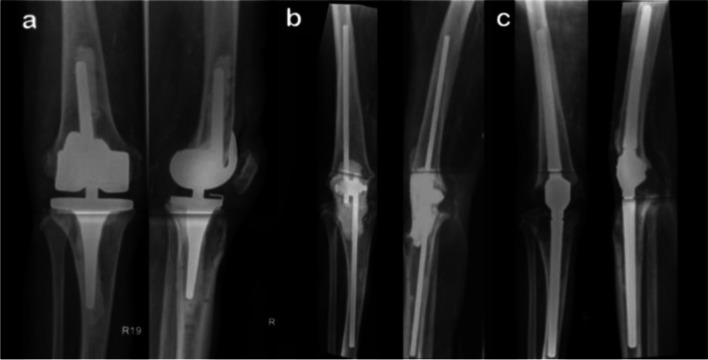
a X-rays of a patient with a hinged cemented total knee arthroplasty. b X-rays after prosthesis explantation, various revision stages and debridements, which led to extensor insufficiency and bone loss, as well as patellectomized with fixed spacer. c The joint reconstruction is carried out by implanting a distance arthrodesis prosthesis (Knee Arthrodesis Module, Brehm, Germany)
